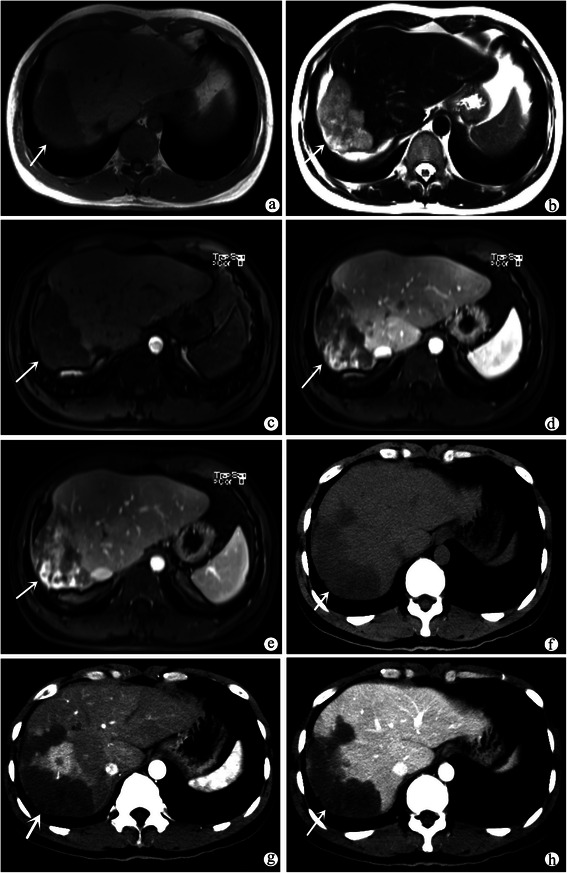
Axial unenhanced MRI scan showed lesions (indicated by the white arrow) were low signal intensity on T1WI (a) and high heterogeneous signal intensity on T2WI (b). The lesions (indicated by the white arrow) showed heterogeneous enhancement in the arterial phase (c), progressive enhancement in portal vein phase and in delayed phase (d and e). Axial CT images (indicated by the white arrow) were similar to MRI images (f, g and h)
